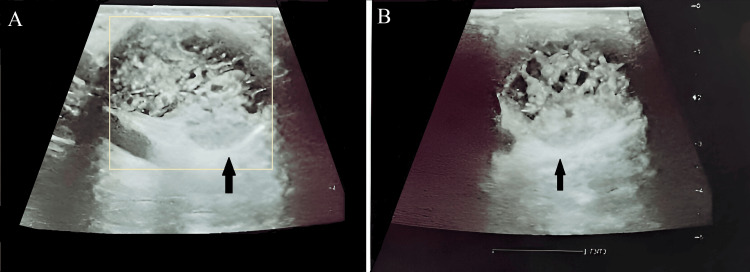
Ultrasound images of the left axilla showing a well-defined, heterogeneous cystic solid lesion with brachial vessels, measuring 37 x 33 x 29 mm (shown in black arrow)
